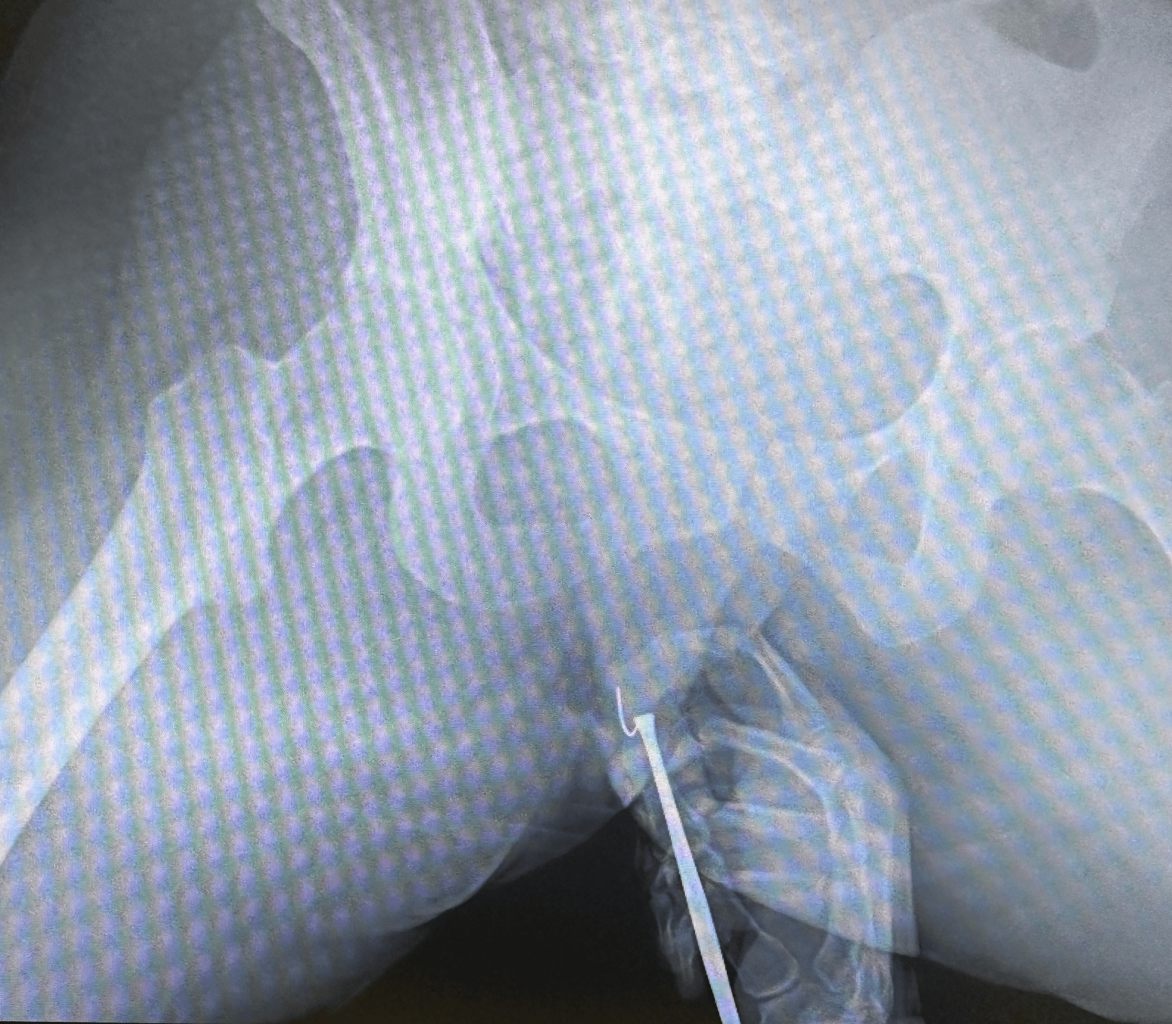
Successful retrieval of the fractured needle fragment. Successful retrieval of the fractured needle fragment following radiographic-guided localization and dissection.
